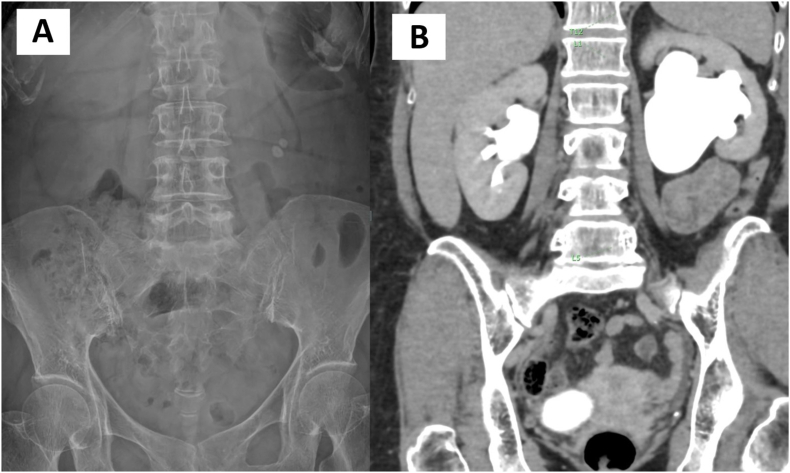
A – Abdominal X-ray showing two left renal pelvic stones; B – Computed tomography scan demonstrating severe left hydronephrosis with recurrent ureteropelvic junction obstruction.
